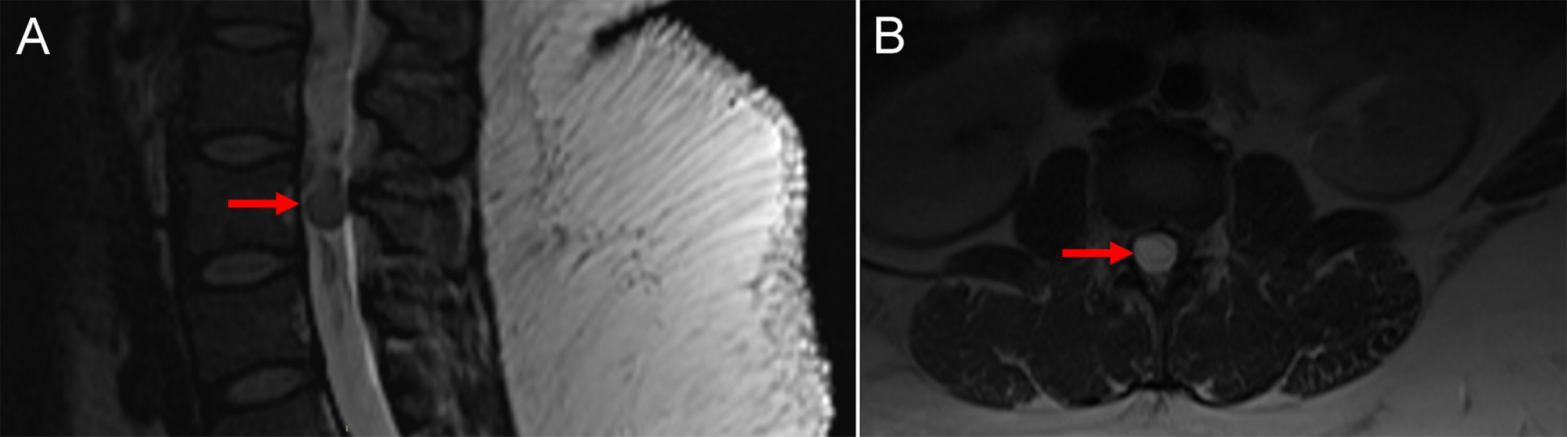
Contrasted lumbar spine MRI A) Diagnostic sagittal MRI of the lumbar spine showing a 4 cm long tumor cyst occupying the entire synovial area of the spinal canal. B) Diagnostic axial MRI at the site of the lesion. The nerve roots are not visible as they are compressed to the side of the lesion.
